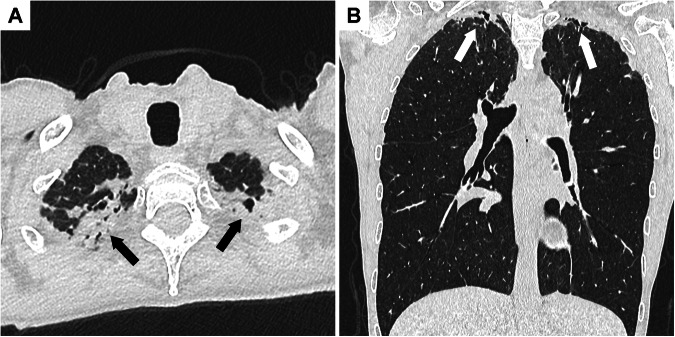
Pleuroparenchymal fibroelastosis in a 70-year-old patient with ulcerative colitis. A CT image in the axial plane shows bilateral subpleural consolidations with bronchiolectasis. B CT image in the coronal plane shows apical distribution and volume loss in the upper lobes. The combination of these CT findings is highly suggestive of the diagnosis of pleuroparenchymal fibroelastosis, and no surgical biopsy has been performed
